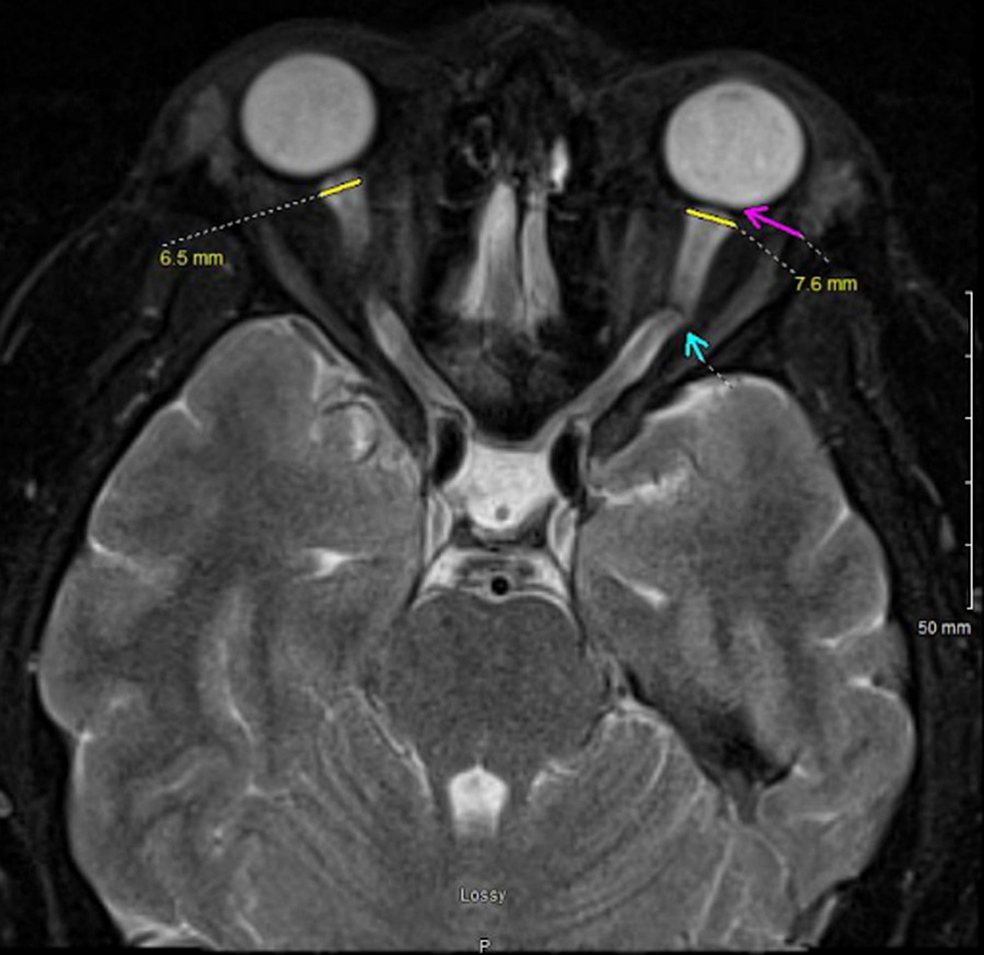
T2-weighted magnetic resonance (MR) image (axial view) showing bilateral expanded optic sheaths (normal range 5.17±1.34 mm to 3.55±0.82 mm) with optic nerve tortuosity (cyan arrow) and posterior globe flattening (magenta arrow), more prominent in the left eye
